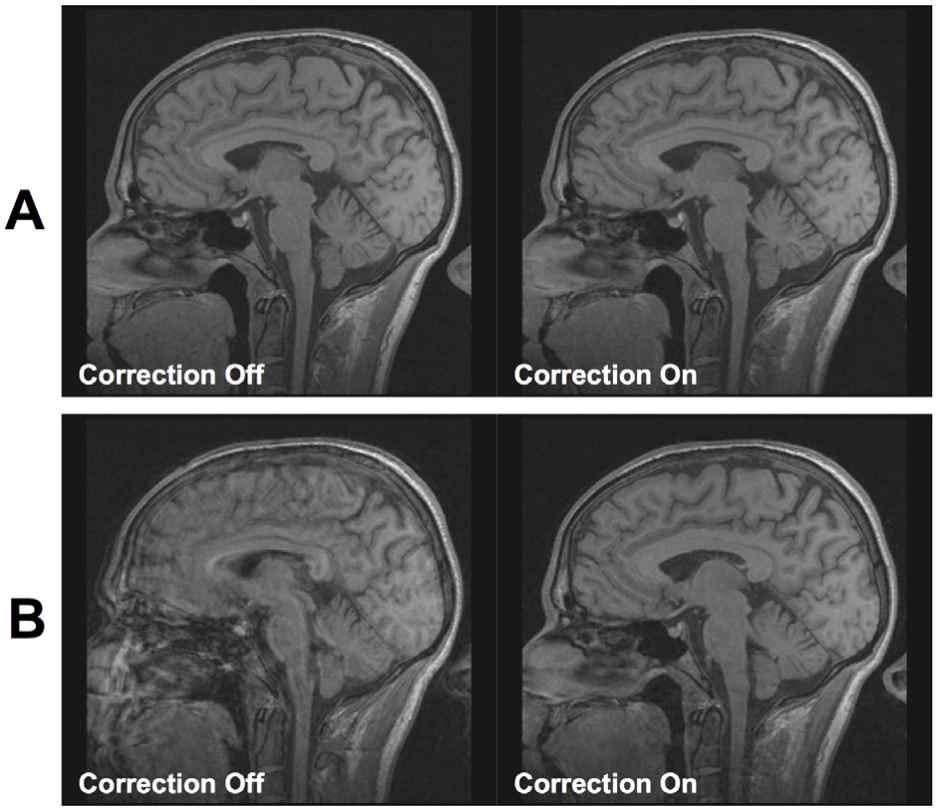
Gradient echo images obtained at 1.5 T, with an in-plane resolution of 1 mm×1 mm and a through-plane resolution of 2 mm. (A) Motion correction applied with no deliberate motion using MR imaging. (B) Motion correction when the subject performed deliberate rotations every 30 seconds during the scan when instructed by the scanner operator (motion range was approximately 8° and 25 mm). At this resolution, motion correction produces a noticeable improvement only in the case of larger movements. See Fig. S1 for a larger version of these images, along with motion plots.
